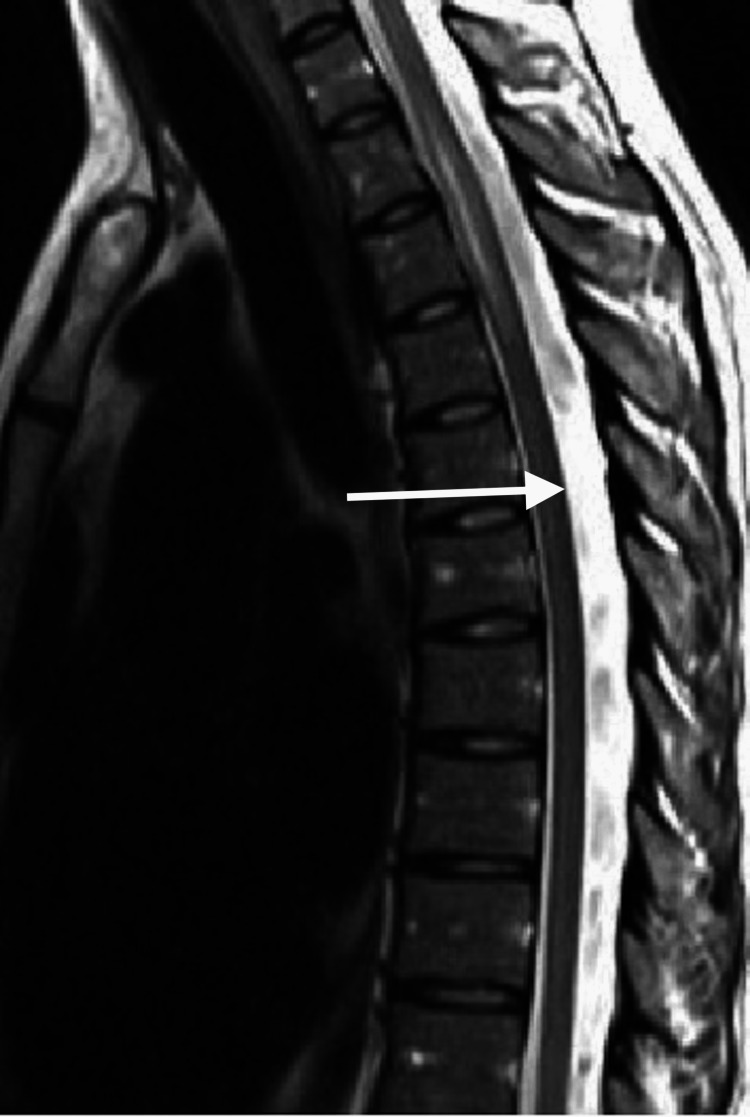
Normal sagittal T1-weighted MRI of the thoracic spine shows epidural fat (white arrow), and it leads to neither any compression nor the displacement of the dural sac MRI: magnetic resonance imaging
